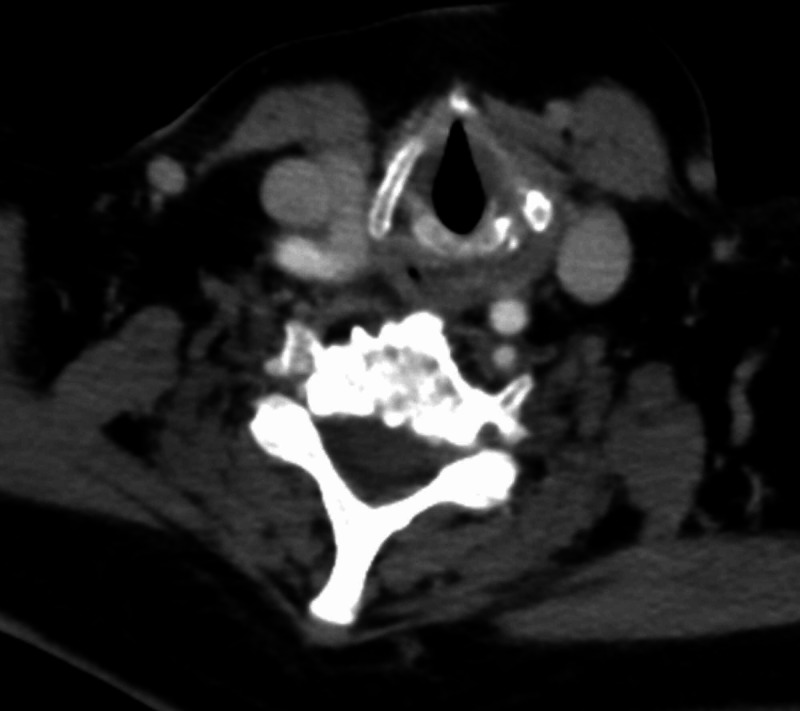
A CT scan with contrast (axial plane) conducted two weeks after the surgery No evidence of the mass is visible in the supraglottic region after the surgical resection.
